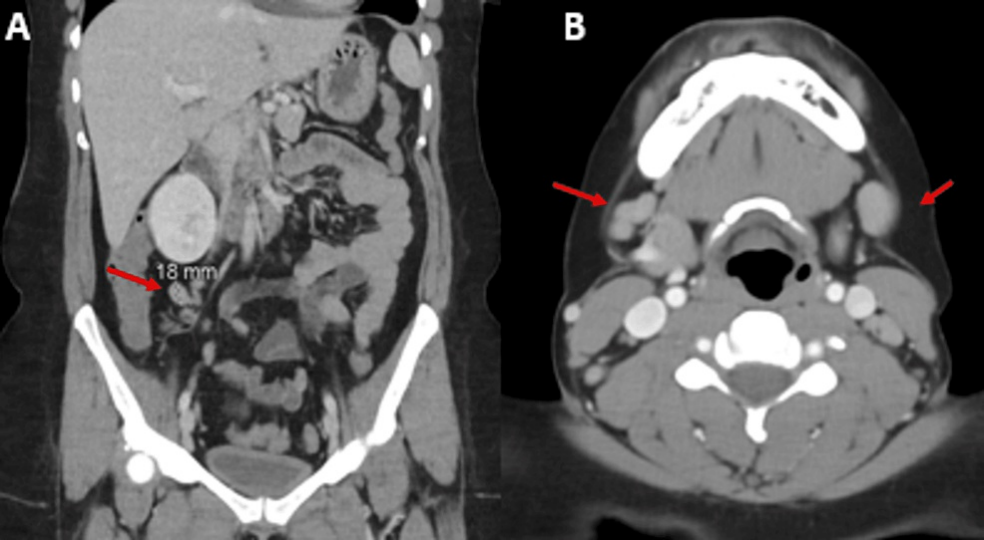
Lymphadenopathy 2A: CT abdomen pelvis with contrast. Increased number of up to 18 mm mesenteric, retroperitoneal, and bilateral lymph nodes. Admission day 4. 2B: CT soft tissue neck with contrast. Bilateral level II and III cervical lymphadenopathies. Bilateral submandibular (level 1B) adenopathy. Left level V adenopathy. Right supraclavicular adenopathy. Admission day 6.
